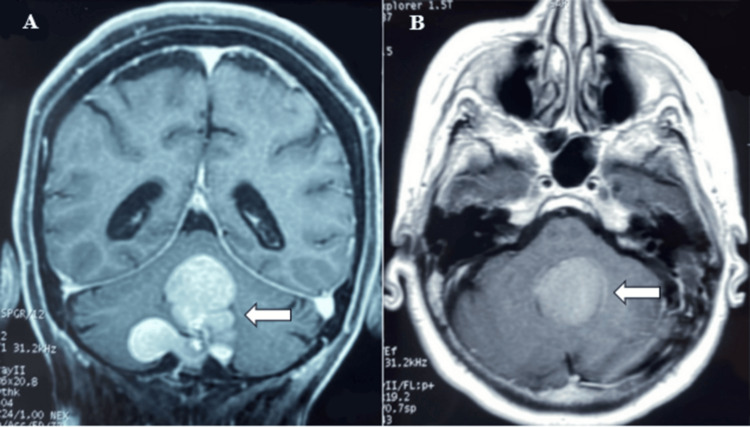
Preoperative contrast-enhanced T1-weighted MRI in coronal (A) and axial (B) views (arrow). The images demonstrate a 4-cm-wide T1-hyperintense multilobulated mass occupying the entire fourth ventricle, with extension through the foramen of Magendie, into the Luschka recesses, and into the bilateral cerebellar hemispheres, more prominently on the right. Moderate ventricular dilatation is also present.
